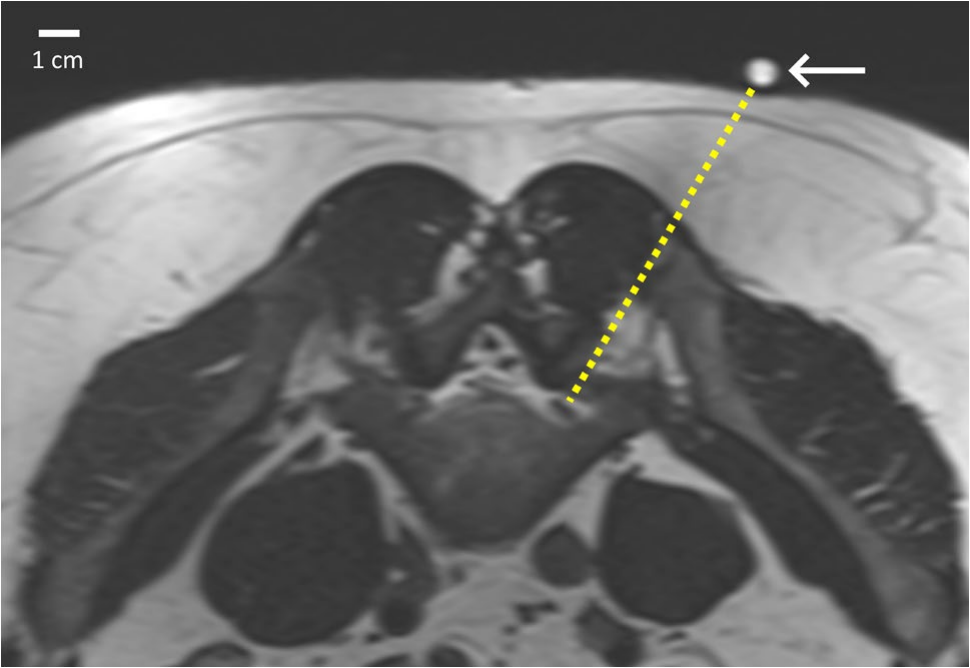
Axial T2-weighted MRI image of 43-year-old man with suspected left L5 radiculopathy in a context of congenital lumbar canal stenosis and L4–L5 discal hernia. A silicon marker (arrow) has been placed on the skin for planification of needle access (dotted line)
